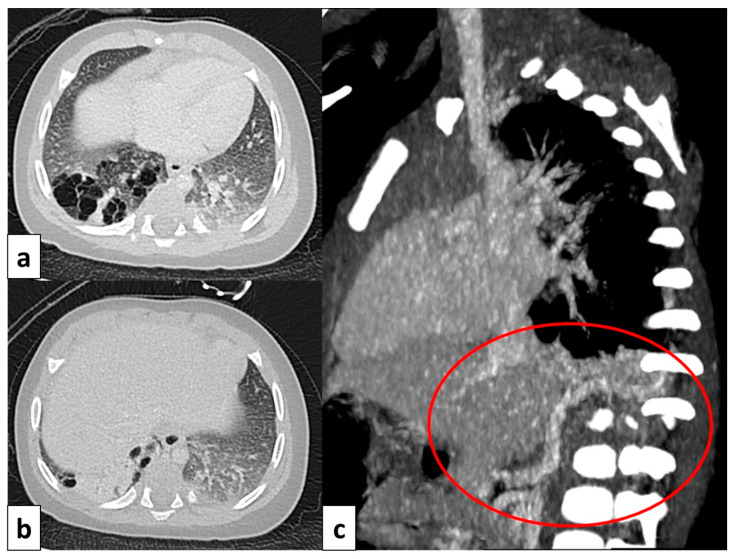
CT image of a 4 month old girl presenting with multiple right cystic lesions <2 cm (a) compatible with CPAM type 2. Parenchymal consolidation (b) associated with the aberrant arterial (c) supply from the celiac trunk (red circle) and venous drainage into the pulmonary venous system, further evaluated with the coronal MiP reconstruction, represent a pulmonary intra-lobar sequestration. The combination of these findings supports the diagnosis of a hybrid lesion.
